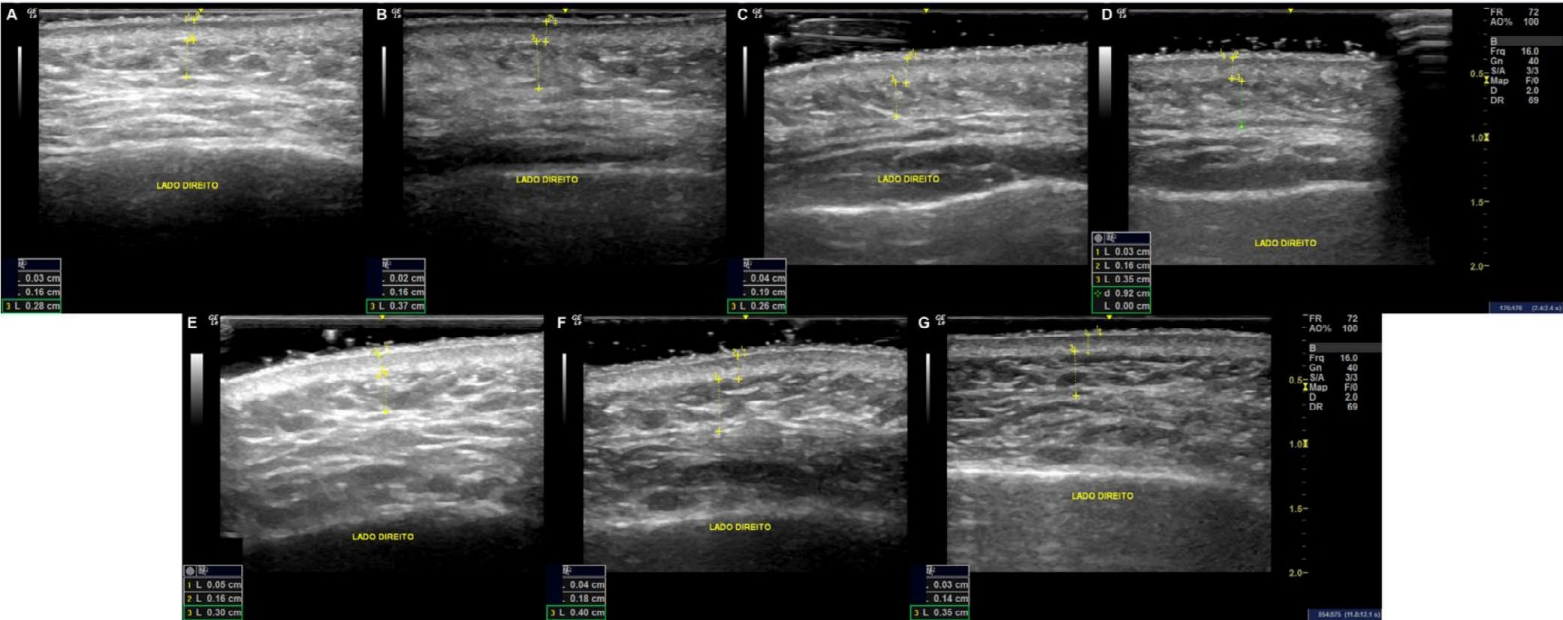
Representative ultrasound images from patients in the CaHA/P (LA/CL) group (A–D) and P (LA/CL) group (E–G), showing structural changes over time. In the CaHA/threads group, images correspond to D–60 (A), D0 (B), D30 (C), and D120 (D). In the threads group, images correspond to D0 (E), D30 (F), and D120 (G).
